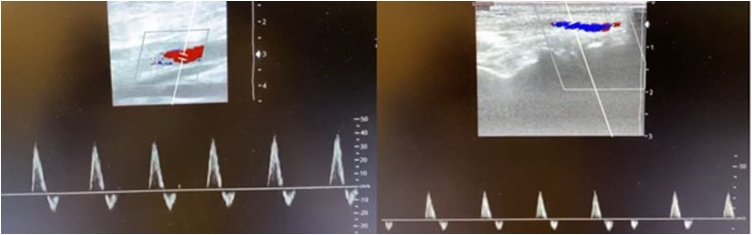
Image showing biphasic flow echo-Doppler in right lower limb arteries.
